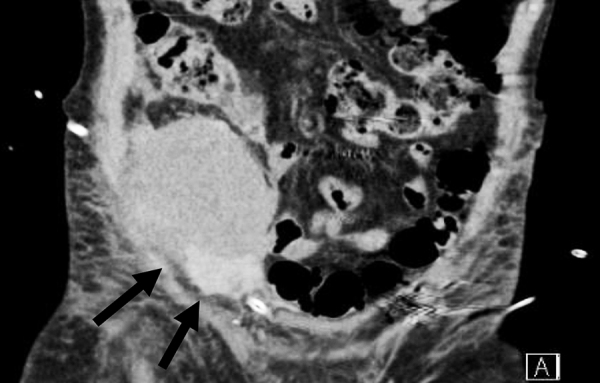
Pelvic computed tomography showing peri-graft hematoma with large diameter of 13 cm (black arrow).
